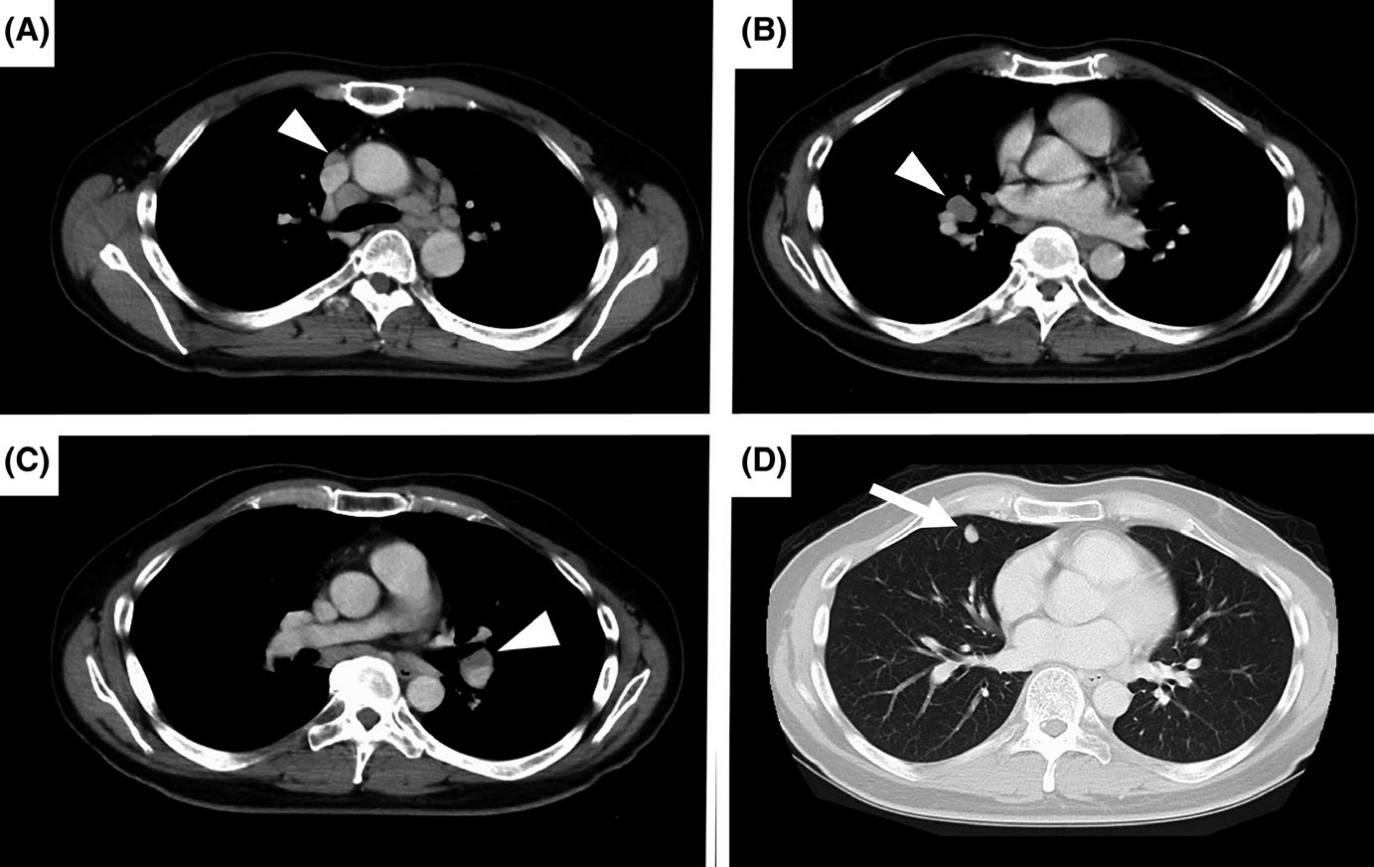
Contrast‐enhanced thoracic computed tomography. (A, B, C) Images of the mediastinal window showing bilateral hilar lymphadenopathy and multiple mediastinal enlarged lymph nodes (arrowheads). (D) Image of the lung‐field window showing a lung nodule in the right S5 region, which was considered to be an intrapulmonary lymph node (arrow)
